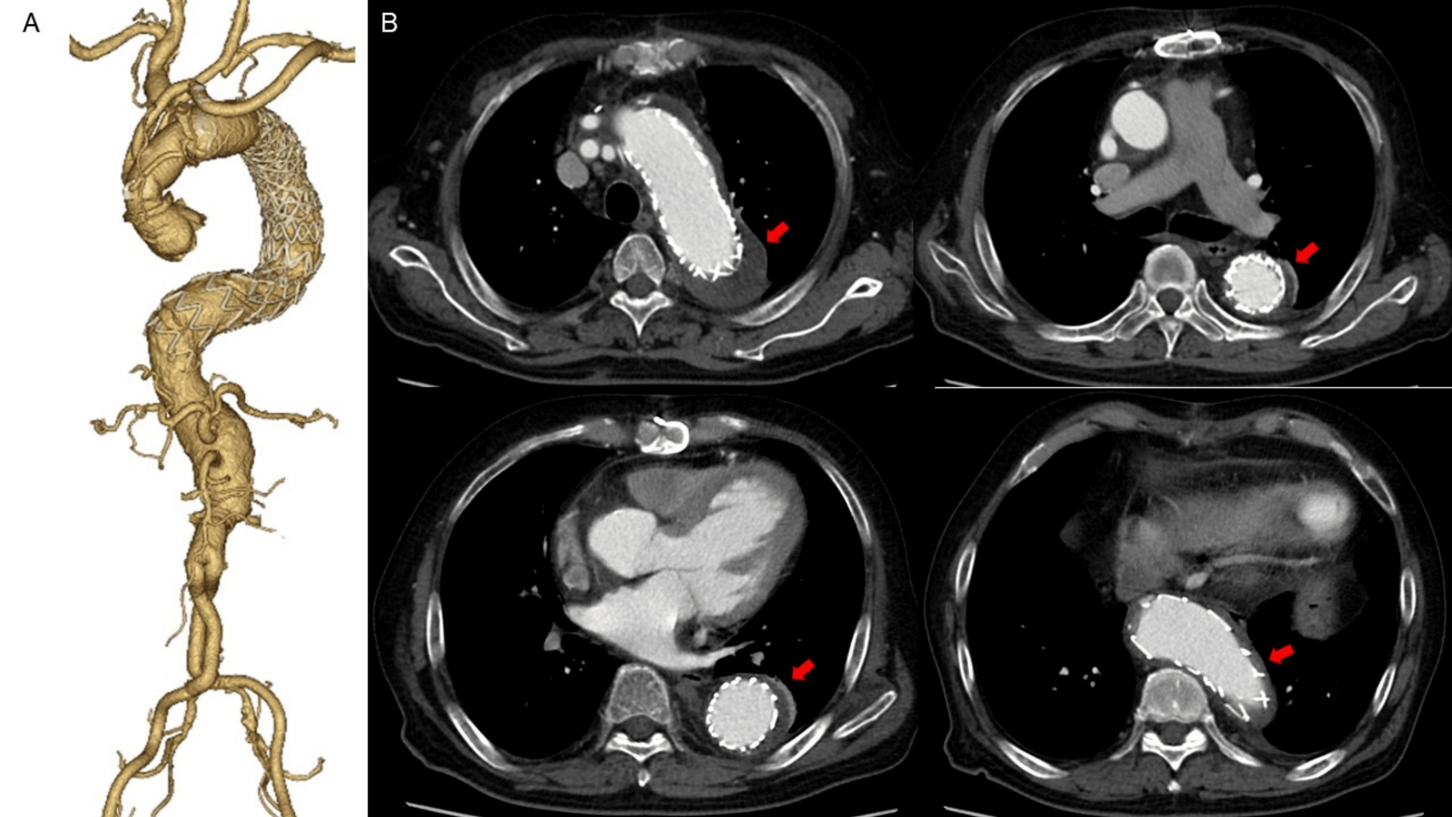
Postoperative aortic contrast-enhanced computed tomography (CT) image showing no endoleak (A) 3D-CT angiography (B) Axial image showed no endoleak (red arrows)
